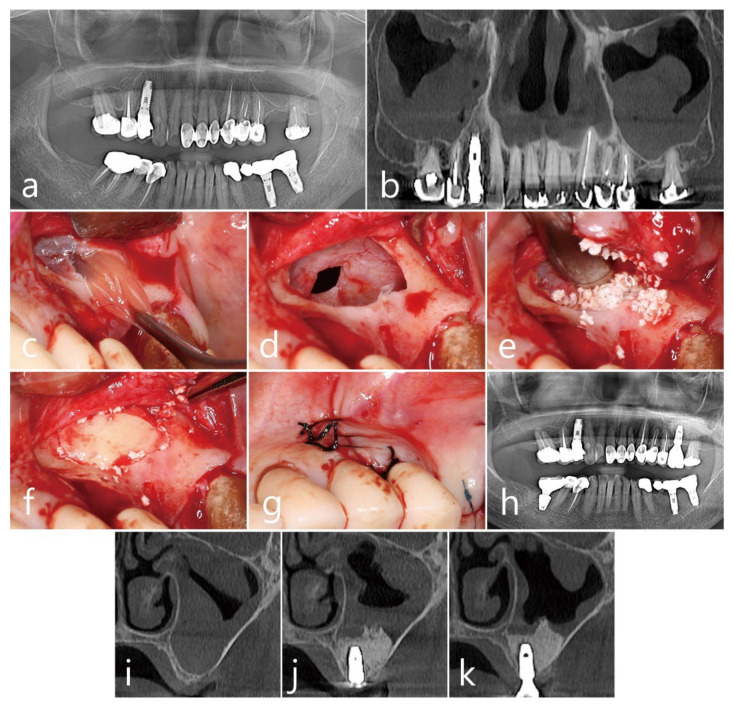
Case 3. Preoperative radiographic features of both maxillary sinuses are shown in the panoramic (a) and CBCT (b) images. The “one-bony-window” technique in left maxillary sinus showing lateral sinus window opening and removal of antral pseudocyst via intentional perforation site (c), sinus floor membrane elevation and reduced size of perforation (d), sinus bone grafting with implant placements (e), repositioning of bony window (f), and primary flap closure (g). Panoramic view obtained immediately after sinus augmentation and implant placement (h). Coronal CBCT images before surgery (i), immediately after surgery (j), and after 1 year of loading (k).
